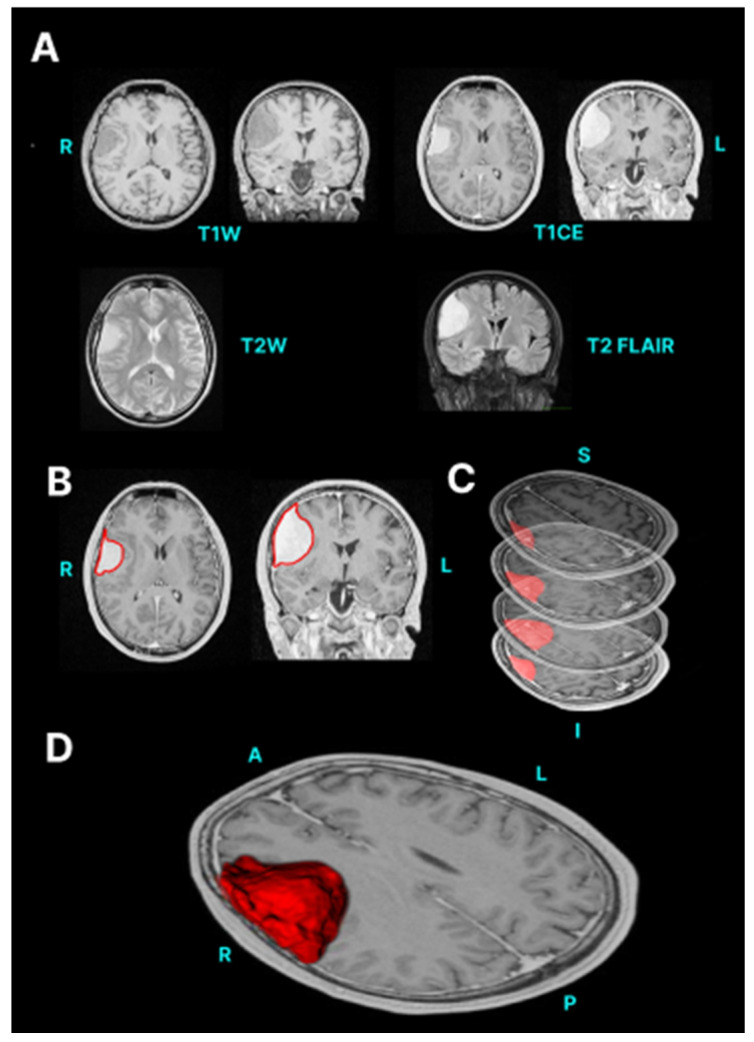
Manual segmentation of a convexity meningioma. (A) Original MRI. (B) Manual delineation of meningioma outline. (C) Interpolation of lesion through various slices. (single column with colour in print).
